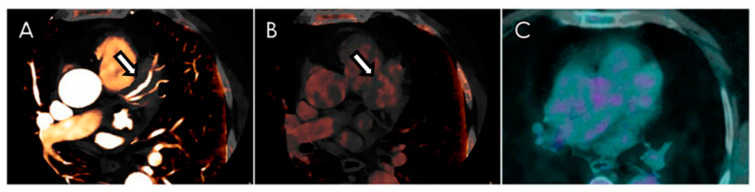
Dual-energy CCTA in a patient with coronary IgG4-RD. Axial iodine map reconstruction does not show any iodine uptake within the pseudo-mass (arrow) during the arterial phase (A), while there was a mild increase of iodine concentration during the delayed phase (B). Similarly, the low-dose PET-CT investigation acquired 60 min after the administration of 18F-FDG did not show a significant increase of the metabolic tracer (C).
